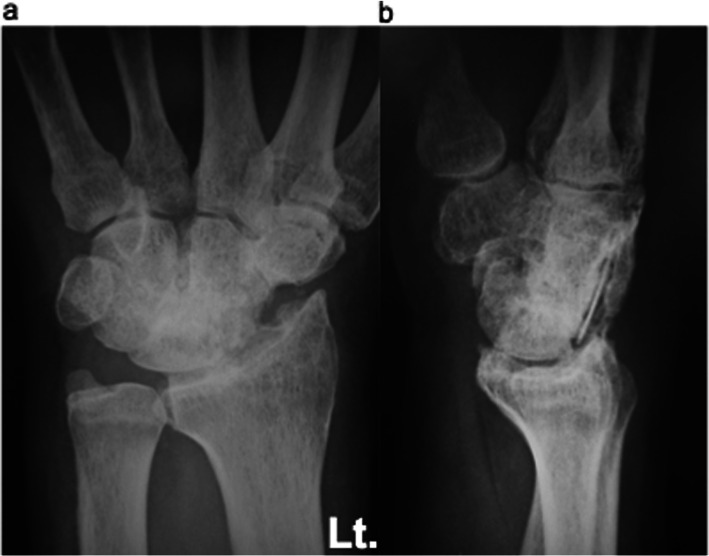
X-ray (a: AP view, b: Lateral view). This shows bone formation occurred onto the BAP 1-year post operatively. Preparing the bone (a) for the inlay of the BAP (b). BAP, bioabsorbable plate
